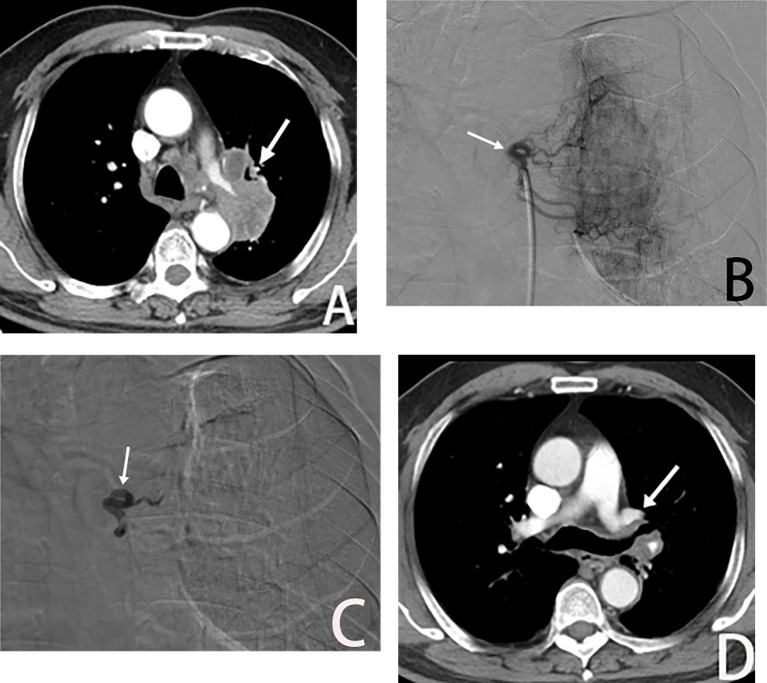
Male, 69 years old, postoperative recurrence of lung adenocarcinoma. (A) The left bronchial artery supplying the left lung tumor (→), BACE was performed with a quality regimen: 200 mg of albumin paclitaxel, 300 mg of carboplatin, and gelatin sponge particles. (B, C) show left bronchial artery arteriogram and embolized (→) on DSA. (D) is CT scan showed significant tumor shrinkage (→), and PR was assessed after 3 weeks of BACE.
